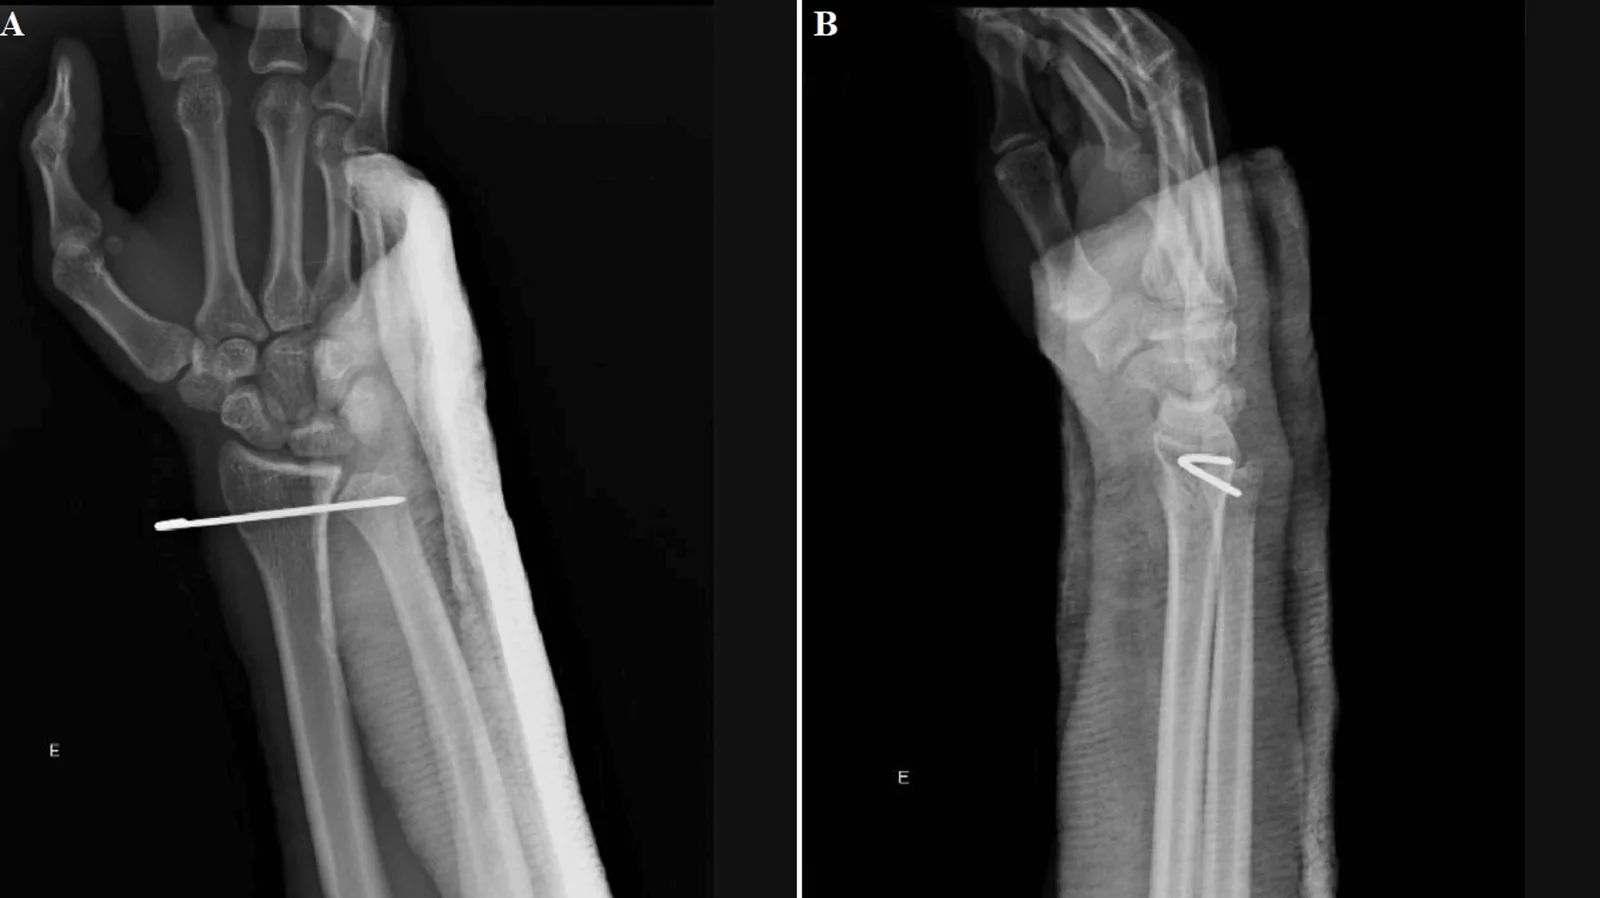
Immediate postoperative radiographs after closed reduction and percutaneous radioulnar transfixation with Kirschner wires. (A) Anteroposterior view. (B) Lateral view.
